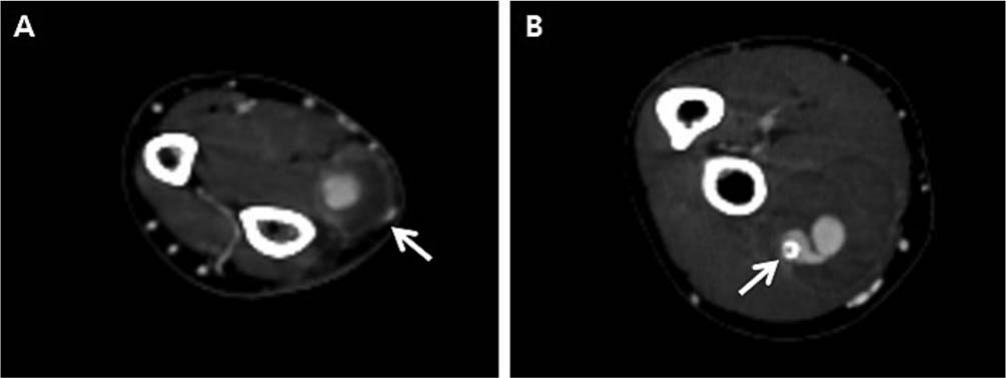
Follow-up computed tomography of the right upper extremity, 2-weeks after the procedure. (A) Pseudoaneurysm at the distal radial artery, size increased from 0.8 cm to 1.5 cm (arrow); (B) Pseudoaneurysm at the proximal radial artery, size increased from 2.0 cm to 4.0 cm with distal migration of covered stent (arrow).
